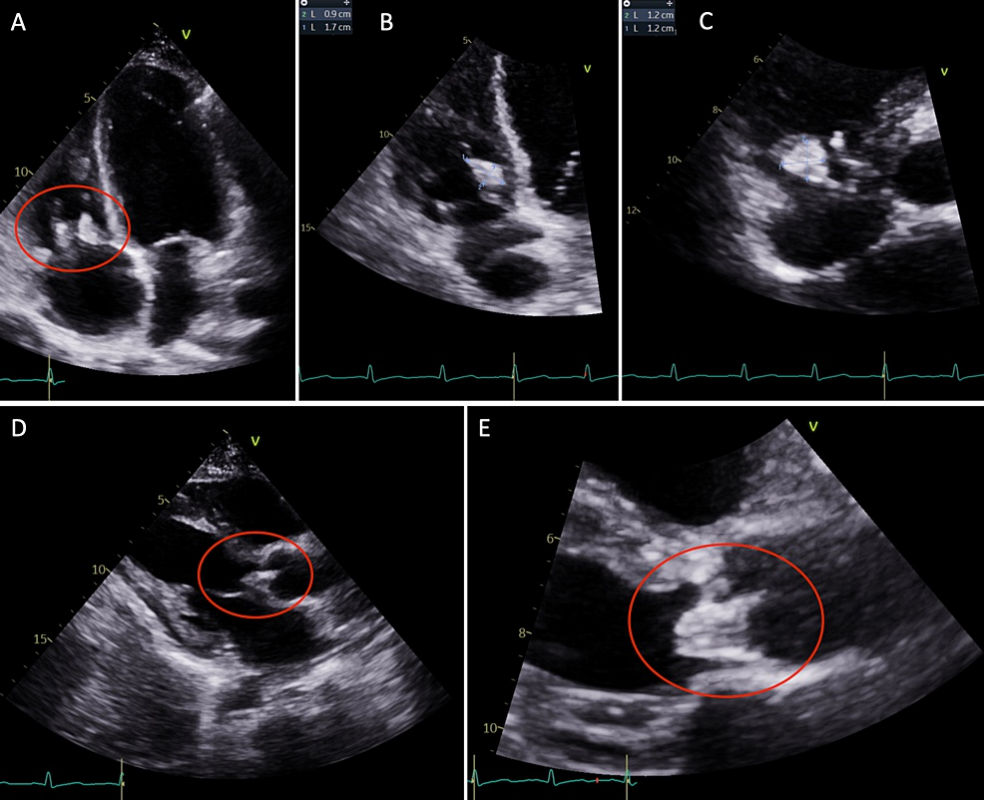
Repeat transthoracic echocardiogram revealing the increasing size of valvular vegetations. Apical four-chamber view (A) and magnified views of the tricuspid valve (B) and (C) showing a 1.2 cm × 1.2 cm echodensity attached to the anterior leaflet and a 0.9 cm × 1.7 cm echodensity attached to the septal leaflet. The parasternal long-axis view (D) and magnified view of the aortic valve (E) showing a 1.7 cm × 1.2 cm echodensity on the noncoronary cusp of the aortic valve.
